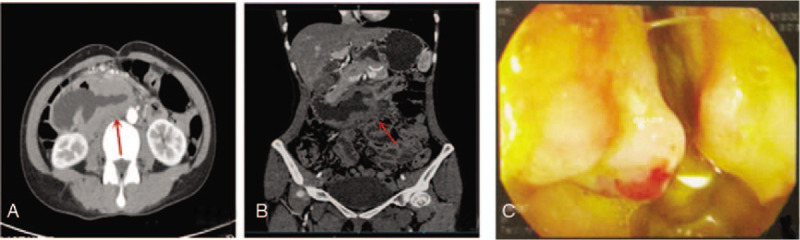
Imaging examinations. Abdominal CT images of transverse section (A) and coronal section (B) revealed space-occupying lesions in the pars horizontalis duodeni (red arrows), bowel-wall thickening, inhomogeneous enhancement, luminal dilatation. C, Gastroduodenoscopy showed irregular protuberant masses in the pars horizontalis duodeni.
